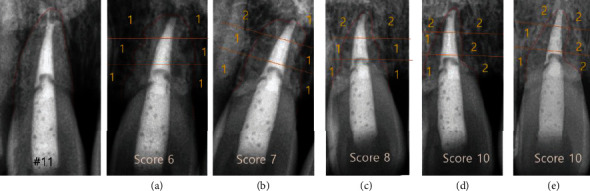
Radiographic index of tooth #11 ((a) 3 years; (b) 4 years; (c) 4 years: 10 m; (d) 5 years: 7 m; (e) 9 years) (Andersson et al.) [14].
